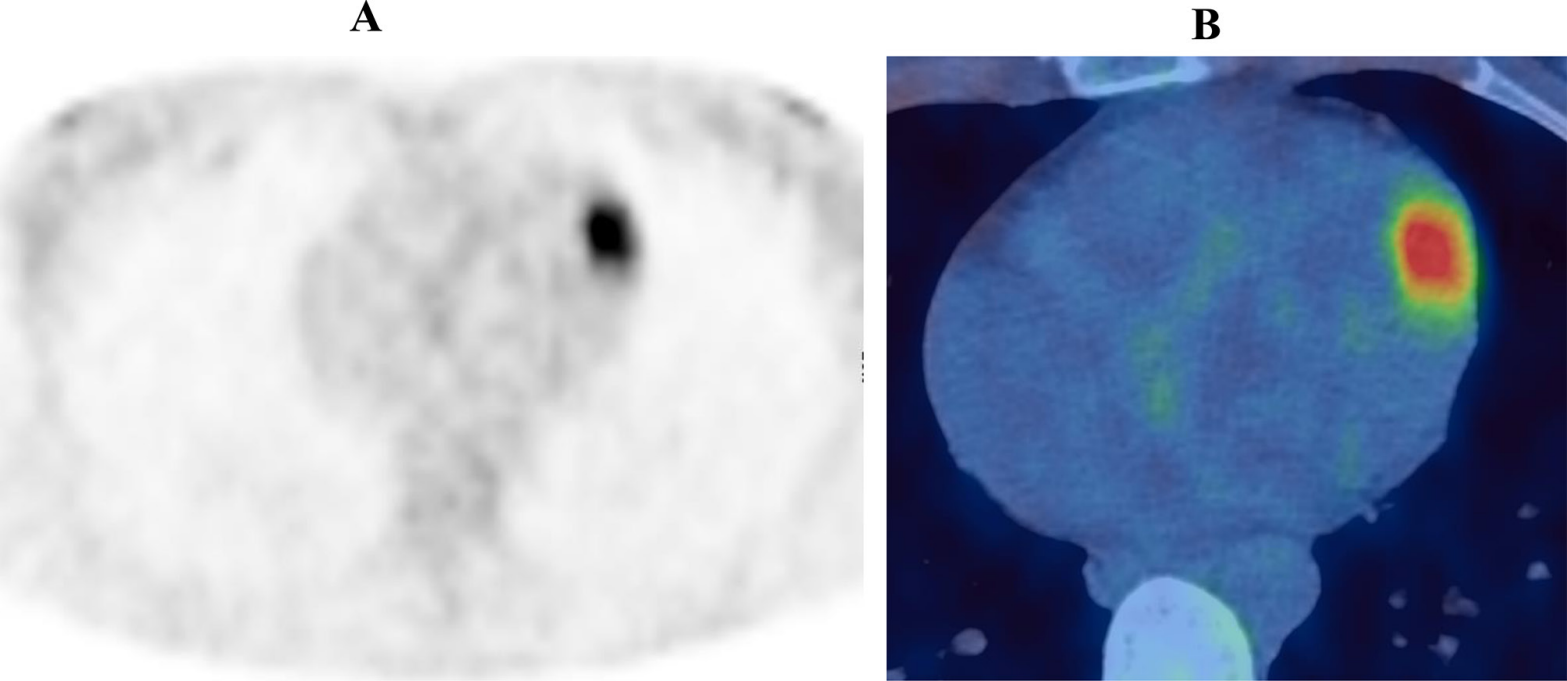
Axial ^18^F-FDG PET only (A) and PET-CT fused (B) images demonstrating the suspicious endocardial (intracardiac) focus of uptake in left ventricle. ^18^F-FDG PET, 18-fludeoxyglucose positron emission tomography.
